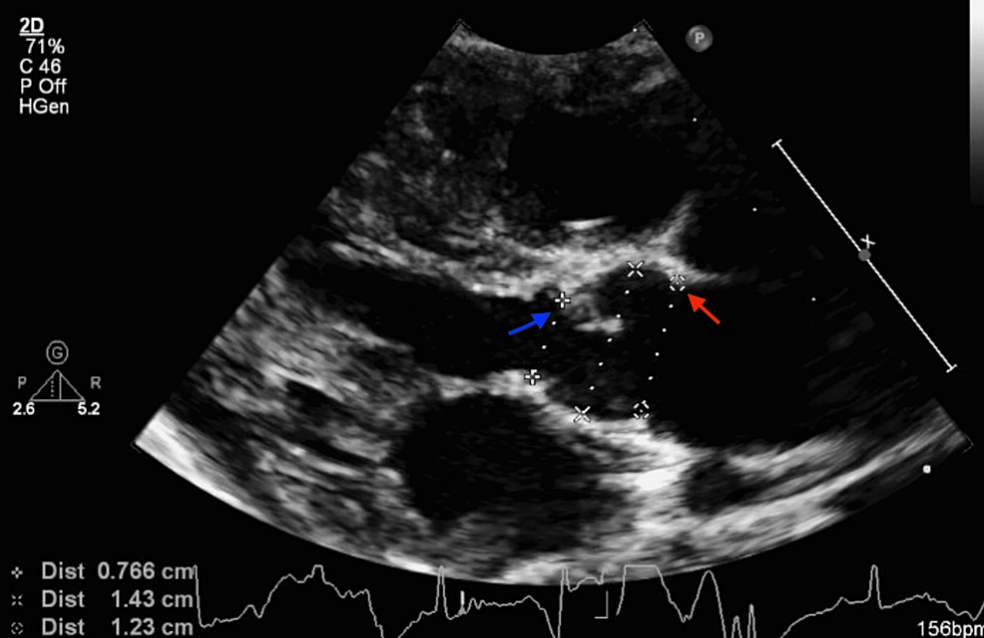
Transthoracic echocardiogram long-axis view showing left ventricular outflow tract, aortic valve area (red arrow), and sub-aortic membrane (blue arrow)
